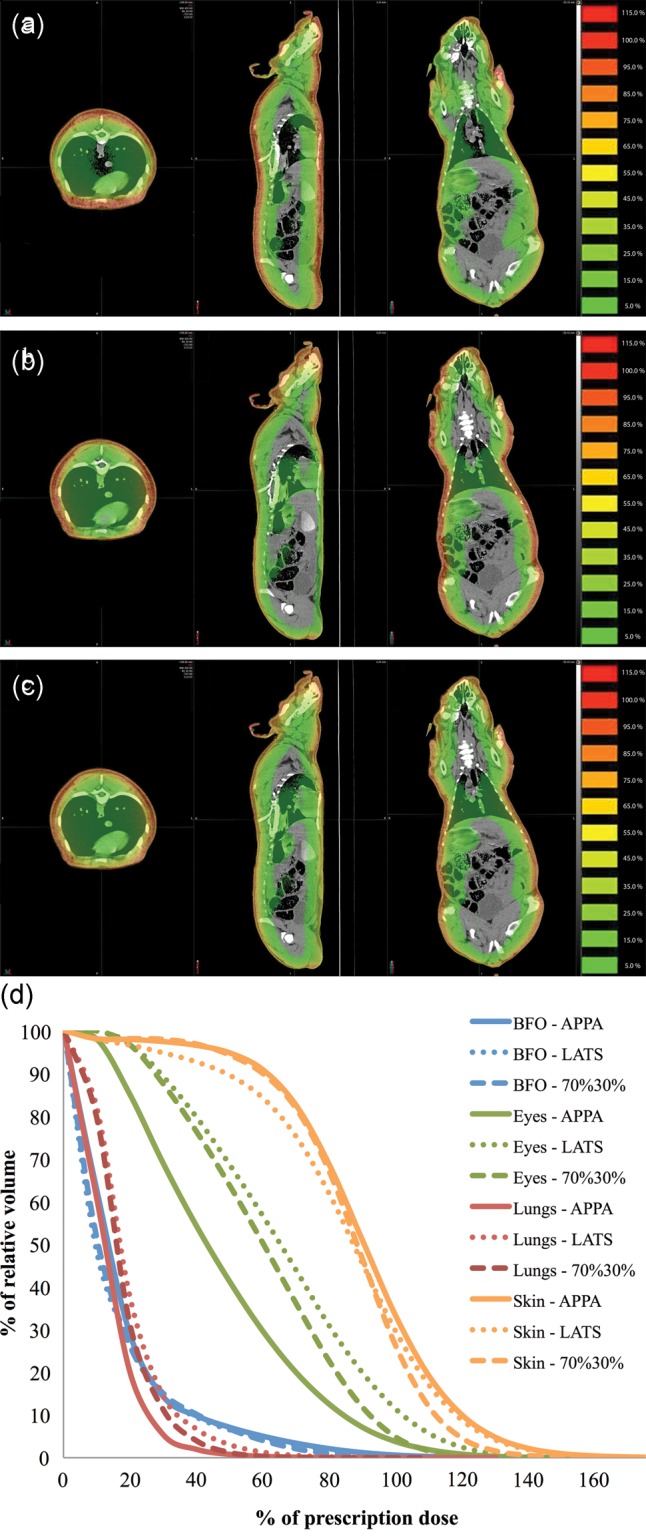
Dose distributions overlaid on Yucatan minipig CT images for 6 + 12 MeV electron irradiations for (a) opposed anterior–posterior beams (APPA), (b) evenly weighted lateral opposed (LATS), and (c) a combination of 70% LATS with 30% APPA beams. The images shown are the transverse, sagittal and coronal planes. (d) DVHs of eyes, lungs, BFO and skin for the three beam orientations.
